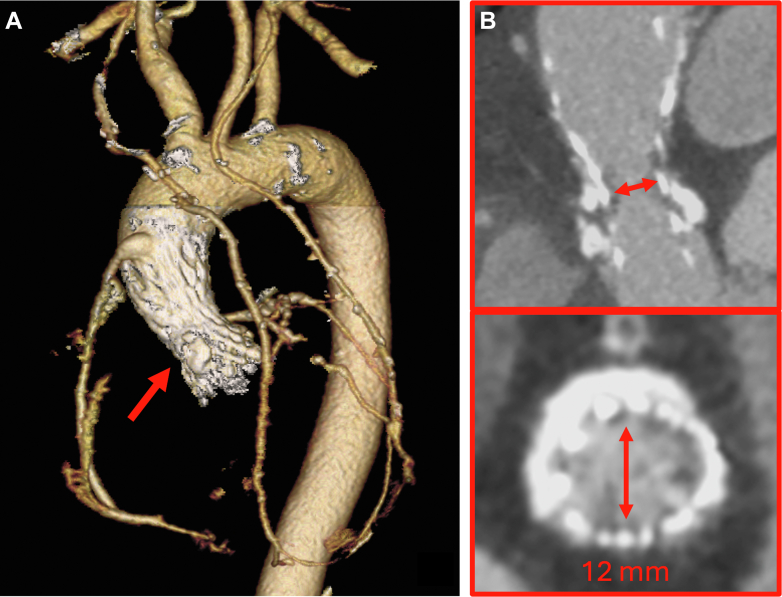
TAVR Failure in Calcified and Small Anatomy Baseline computed tomography showed coronary graft patency and an extremely small residual aortic root area after transcatheter aortic valve replacement (TAVR) (A), especially at the level of the sinotubular junction resulting in approximately 140 mm^2^ and a maximum diameter of 12 mm (red arrows) (B).
